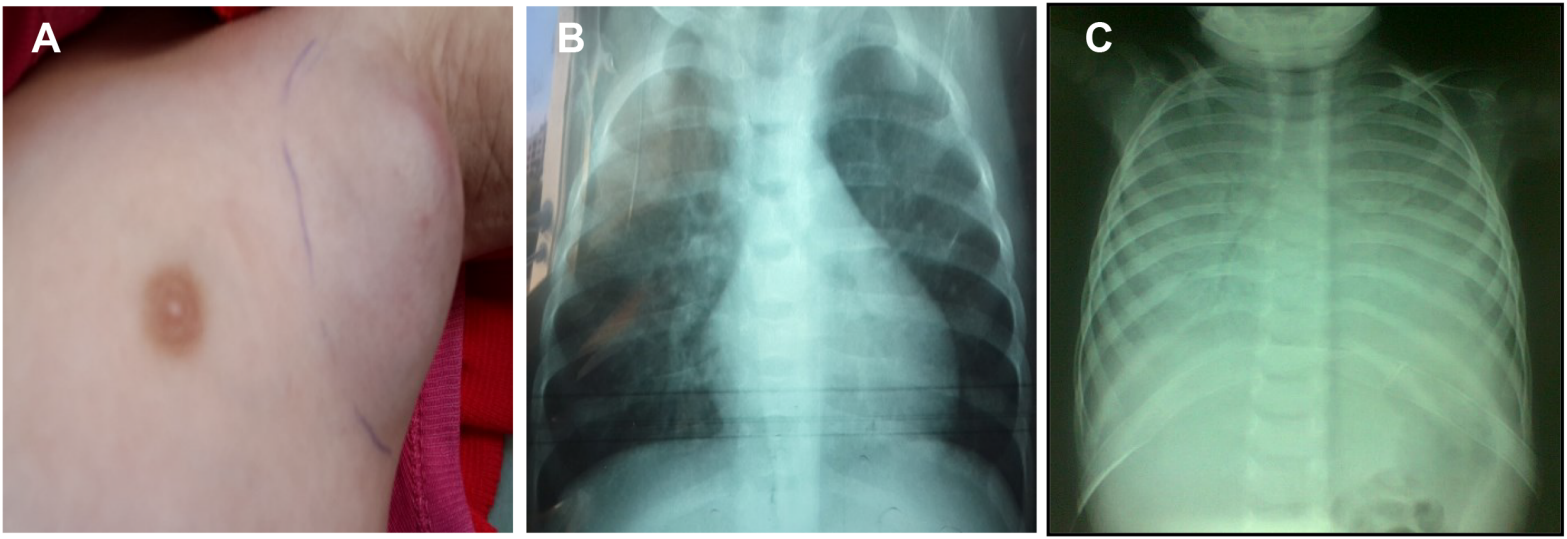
Clinical and radiological findings in Patient 2. (A) Left axillary lymphadenopathy consistent with locoregional BCGitis. (B) Baseline chest radiograph showing perihilar and bronchial interstitial markings. (C) Follow-up chest radiograph demonstrating diffuse bilateral pulmonary opacification consistent with CMV pneumonitis.
